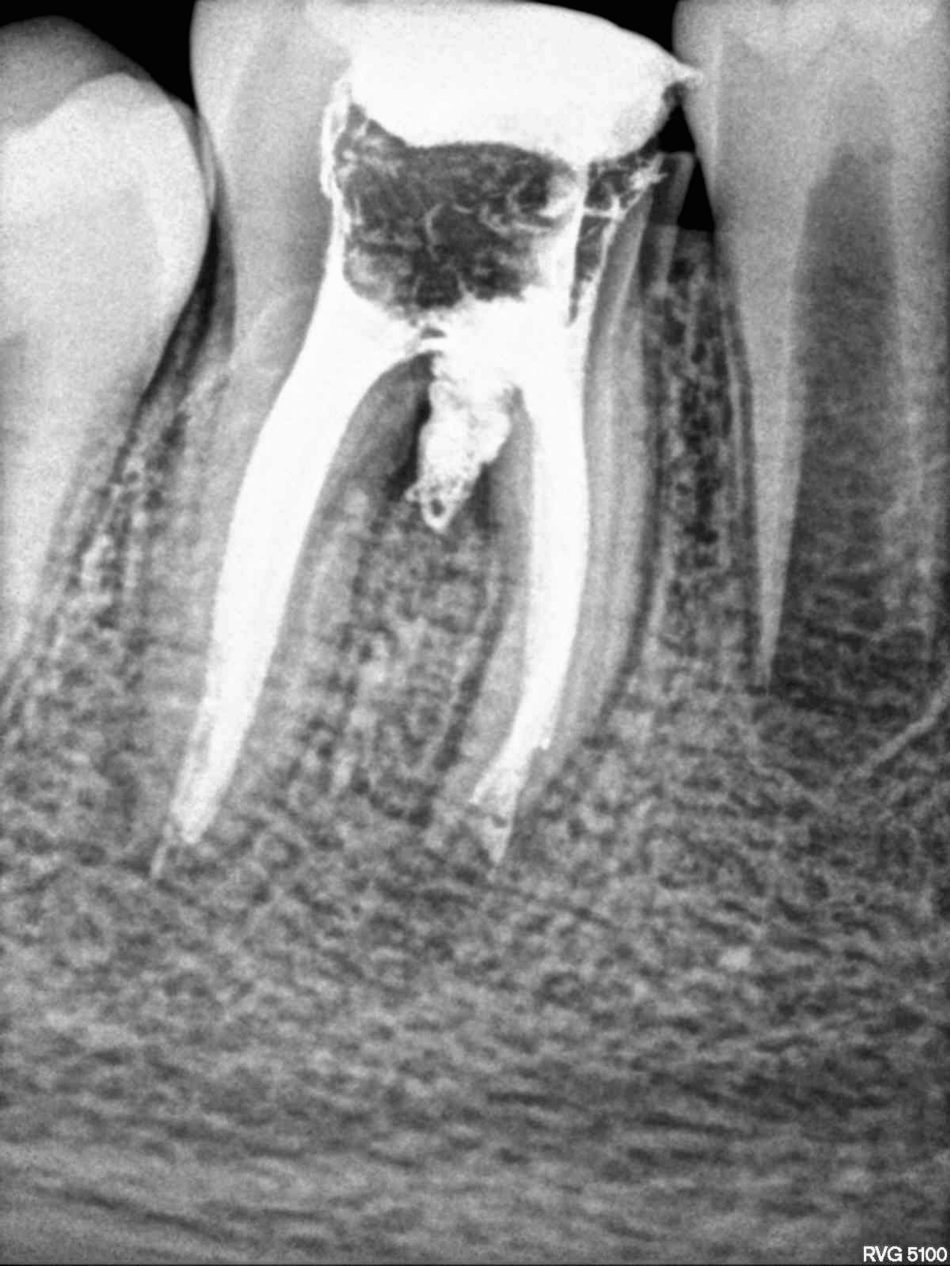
Post-obturation intraoral periapical (IOPA) radiograph with respect to 46
